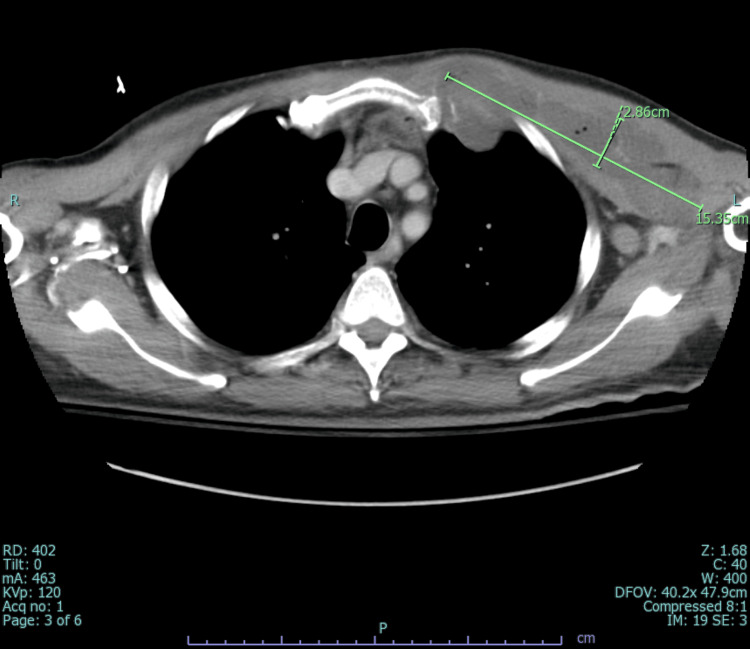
Computed tomography scan (axial view) showing an intramuscular abscess in the left pectoralis muscle.
